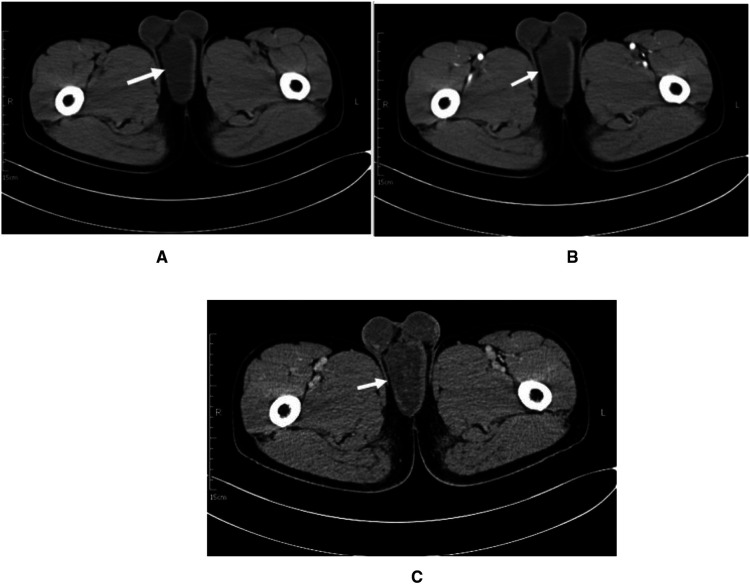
Patient imaging data. (A) CT plain axial image.(B) Arterial phase of the contrast. (C) Venous phase of the contrast.
